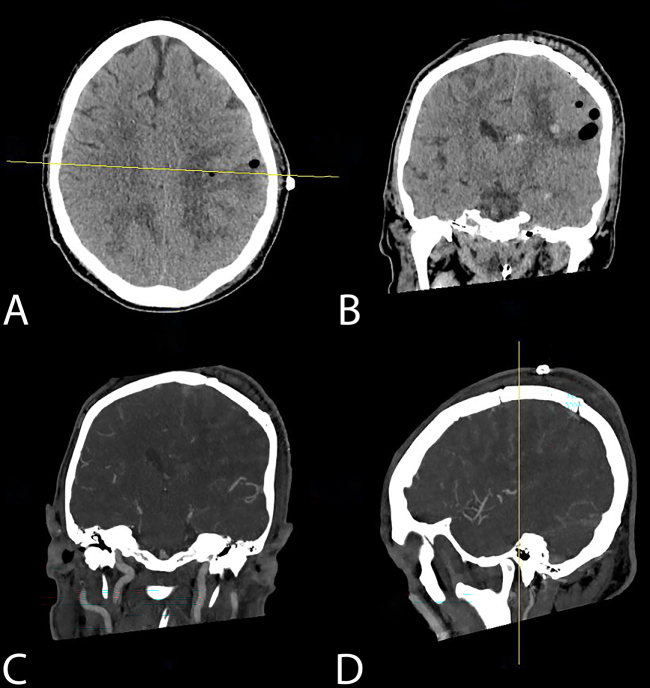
Post-operative non-contrast CT brain – axial (A), coronal (B) views, and post-operative CT brain angiogram – coronal (C), sagittal (D) views.
